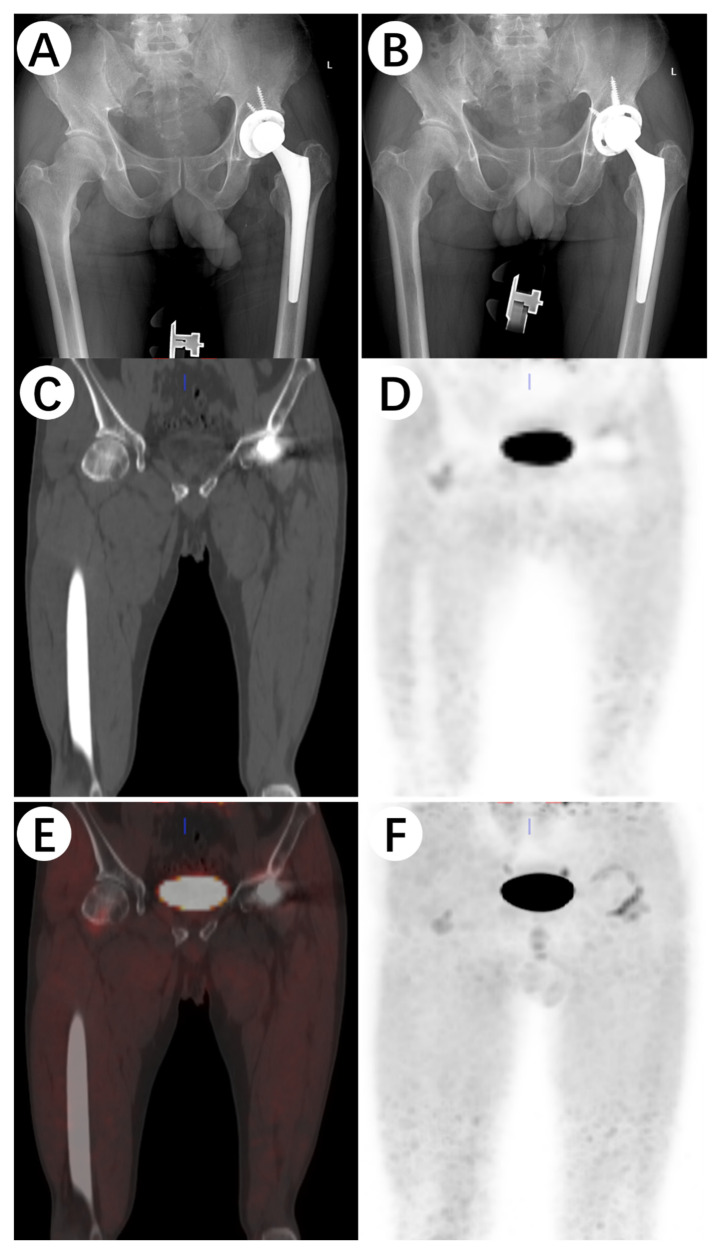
A 56-year-old male patient presented with persistent left hip pain for approximately one year following a total hip arthroplasty (THA), which had been performed following a femoral neck fracture 3.5 years ago. Initial X-ray (A) demonstrated that the abduction and anteversion angles seemed not ideal. No obvious joint space narrowing or osteophyte formation was observed in the contralateral (right) hip, corresponding to a Kellgren–Lawrence (K–L) grade of 2. The patient subsequently underwent ^68^Ga-FAPI PET/CT (uMI 510, United Imaging Healthcare, Shanghai, China). Patients were not required to fast before imaging. PET/CT was performed 1 h after intravenous administration of ^68^Ga-FAPI (1.8–2.4 MBq/kg), with an acquisition time of 4 min per bed position. Low-dose CT (120 kV, 30–50 mAs) was used for attenuation correction and anatomical localization, and PET images were reconstructed using a standard ordered-subset expectation maximization algorithm. Through ^68^Ga-FAPI PET/CT (this test was approved by the Ethics Committee of our hospital and registered at the Chinese Trial Registry: ChiCTR2000041204, and written informed consent was obtained from the patient for the purpose of scientific research), obvious linear uptake along the acetabular prosthesis and high uptake at the junction of the head and neck were observed (C–F). According to our previous report about the diagnostic classification of ^68^Ga-FAPI in pain after hip replacement, this patient was diagnosed with acetabular prosthesis loosening [1], and during revision surgery, the acetabular prosthesis was not stable and replaced. Notably, before revision surgery, mildly increased ^68^Ga-FAPI uptake was already present at the right femoral head–neck junction (SUVmax = 3.87), despite the absence of corresponding radiographic abnormalities. Half a year after revision, the patient returned to our hospital and complained about severe pain in the right hip. Follow-up X-ray (B) again showed no definite joint space narrowing or osteophyte formation, corresponding to a K–L grade of 2; however, subtle surface irregularity of the right femoral head cartilage was observed compared with the pre-revision radiograph. Initial laboratory tests were mildly abnormal, with the results of C-reactive protein (mg/dL) and ESR (mm/h) being 1.272 and 69, respectively.
